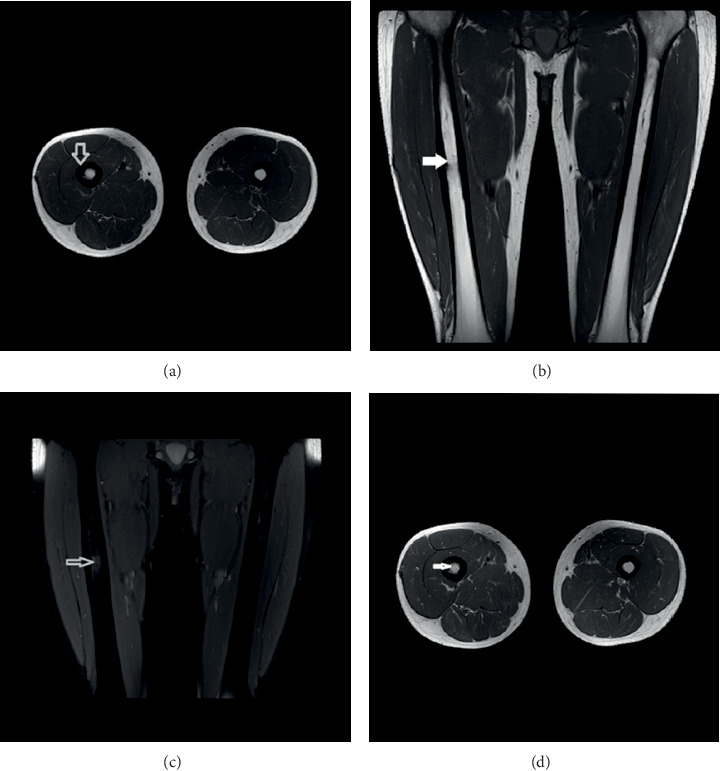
MRI scan at 48 months post-RFA. Axial (a) and coronal (b) T1WI images show no bone oedema (white arrow) and only minor cortical thickening (arrowhead). Proton density WI (PDWI) with fat suppression MRI scan image (c) verified the absence of bone oedema (grey arrow). Axial T1WI MRI scan image (d) following the administration of contrast media suppression show no enhancement.
